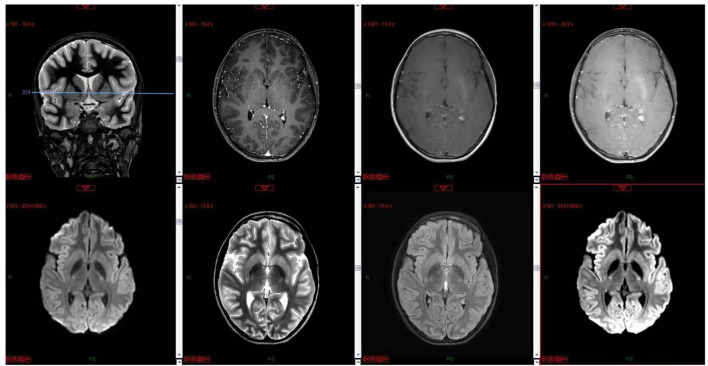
MRI done on day 9 following the CO poisoning and 3 days after the onset of seizures. The MRI of the basal ganglia in a single coronal localizer image and multiple axial images including T1 pre- and post-contrast, diffusion, FLAIR, T2 at the level of the basal ganglia show normal signal within the basal ganglia and deep nuclei.
